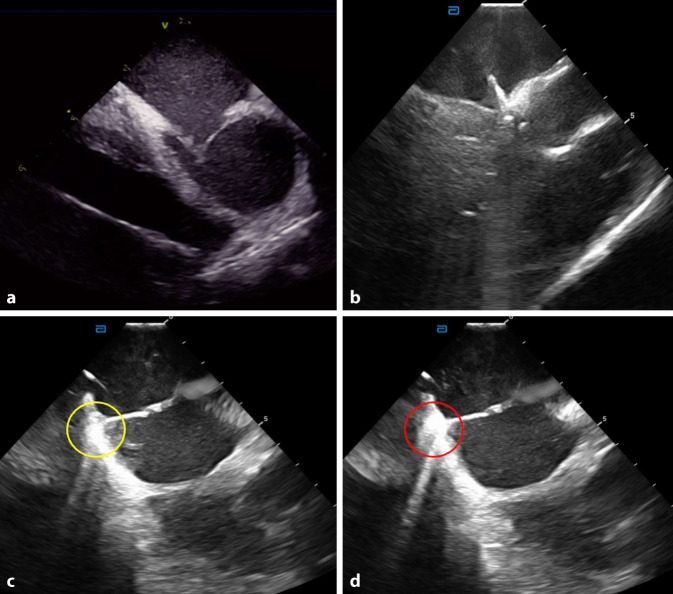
a A 6-year-old girl with left-sided atrioventricular tachycardia. Transeptal puncture performed with intracardiac echocardiography. The tip of the Brockenbrough needle is tenting against a floppy intra-atrial septum only 4 mm away from the posterior wall of the left atrium. b A 12-year-old boy with a parahisian accessory pathway ablated with a cryoablation catheter positioned in the His region. c A 13-year-old boy with right posterolateral focal atrial tachycardia with the ablation catheter in contact with the myocardium during radiofrequency (RF) ablation. The yellow circle shows myocardial echogenicity at the beginning of RF application. d The myocardium in contact with the RF catheter suddenly becomes brighter and larger, corresponding to the oedema caused by RF application
